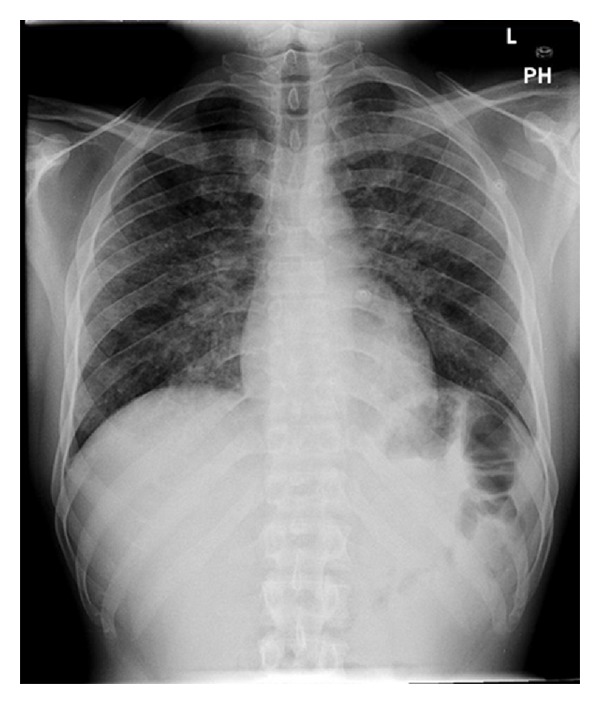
Upright posterior-anterior projection chest X-ray demonstrating diffuse reticular-nodular opacities in both lungs.
